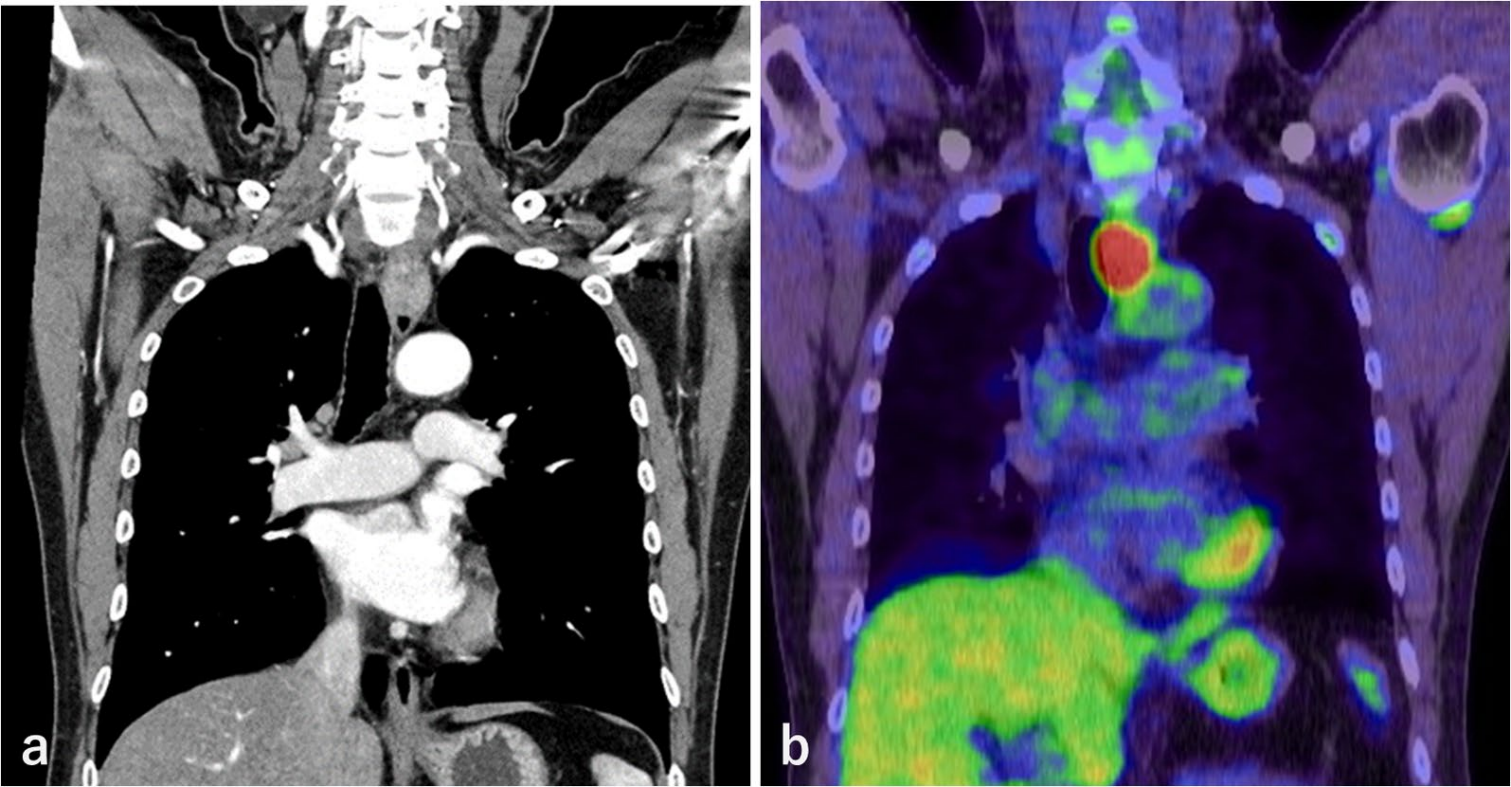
Contrast-enhanced CT and FDG–PET/CT images. a Contrast-enhanced CT showed enhanced wall thickening in the cervicothoracic esophagus that displaced the trachea to the right side. b FDG–PET/CT showed high FDG uptake with a maximum SUV of 21.6 only in the primary lesion
